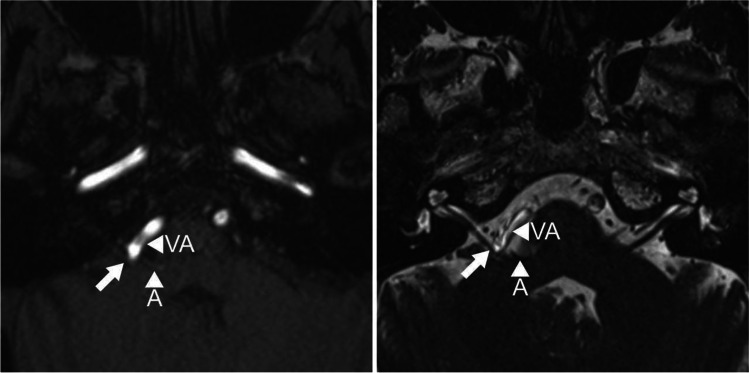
Preoperative MR imaging with TOF (left) and CISS (right) axial images showing a compression of the facial nerve root exit zone (arrow) by AICA (A) and VA (VA)
